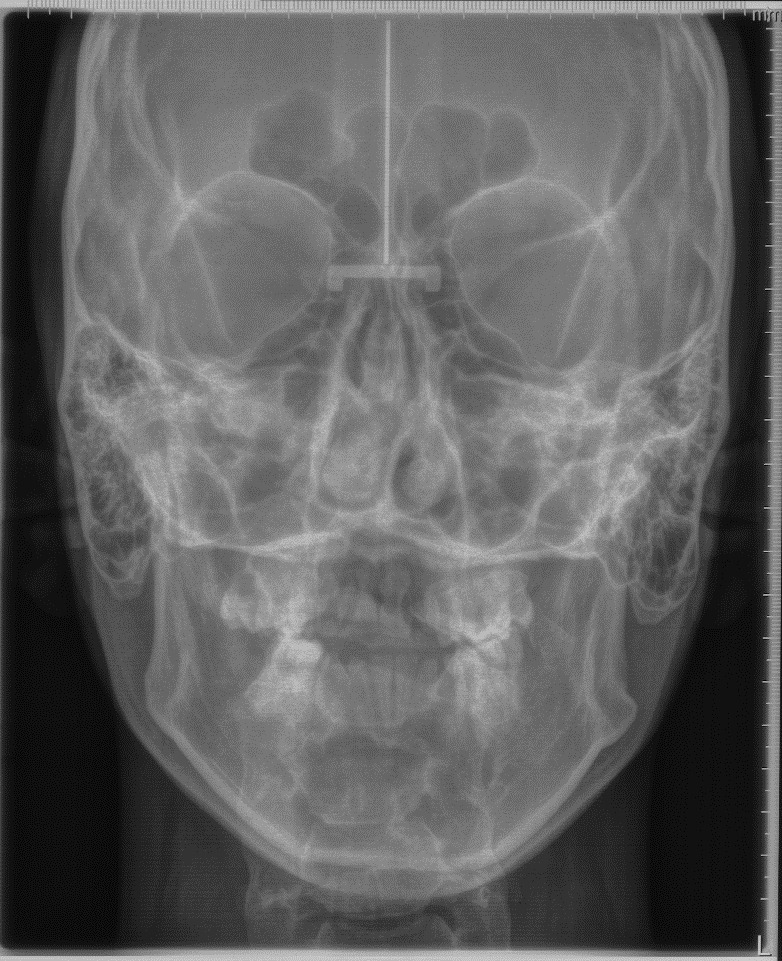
Pre-treatment posterior anterior (P-A) view radiograph of the head.
